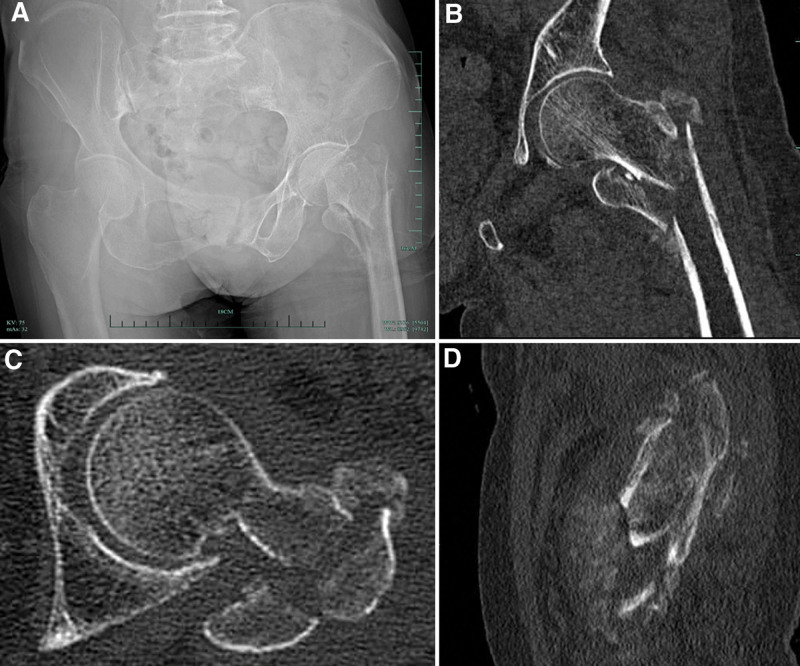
Preoperative imaging findings of the patient. Radiographs (A), coronal (B), transverse (C), and sagittal (D). CT scans identified a left intertrochanteric fracture accompanied by greater and lesser trochanteric fractures with varus deformity, and callus growth at the fracture end. CT = computed tomography.
